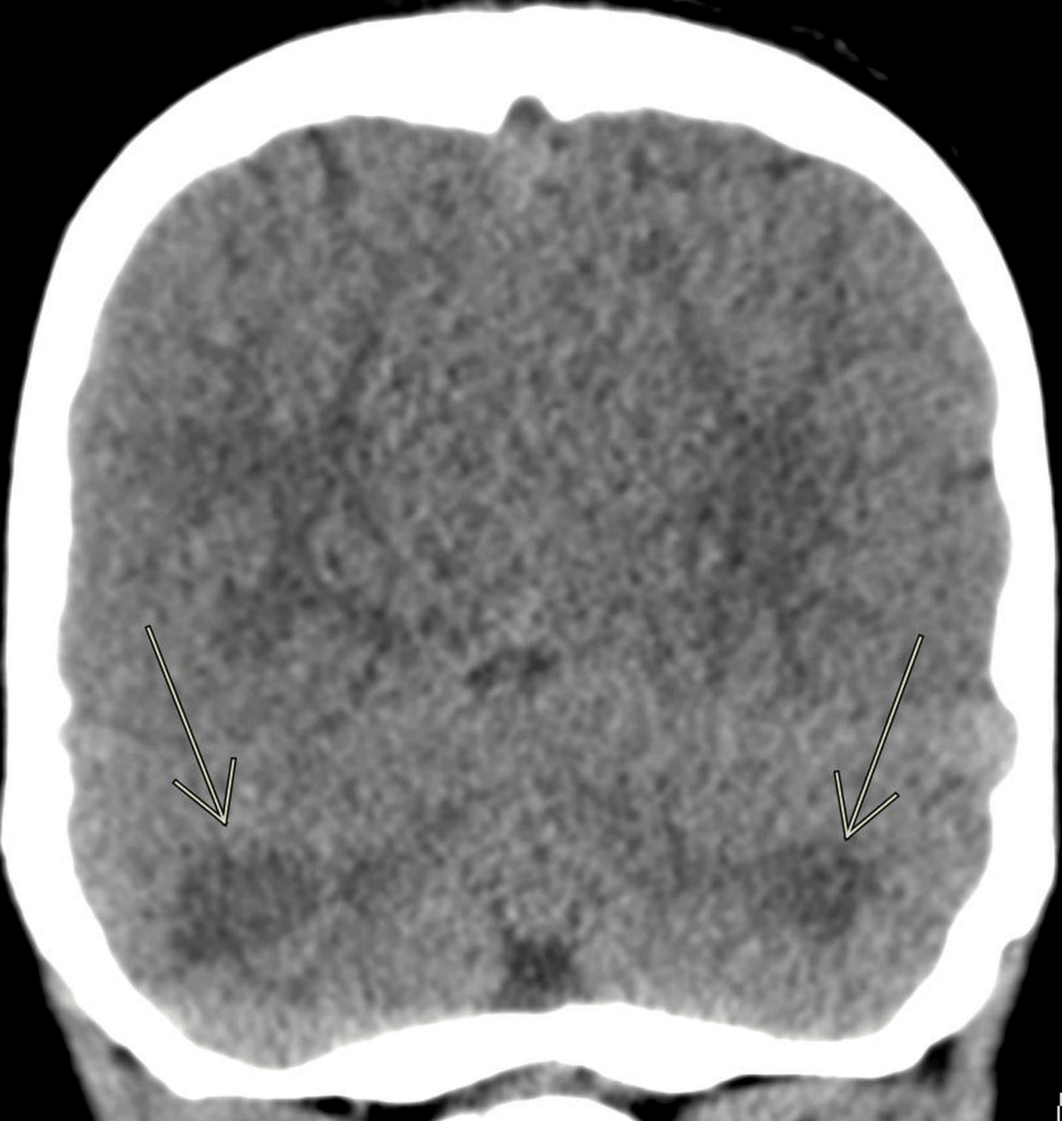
CT head Coronal CT obtained on the day of the presentation shows diffuse cerebellar white matter hypodensity.
